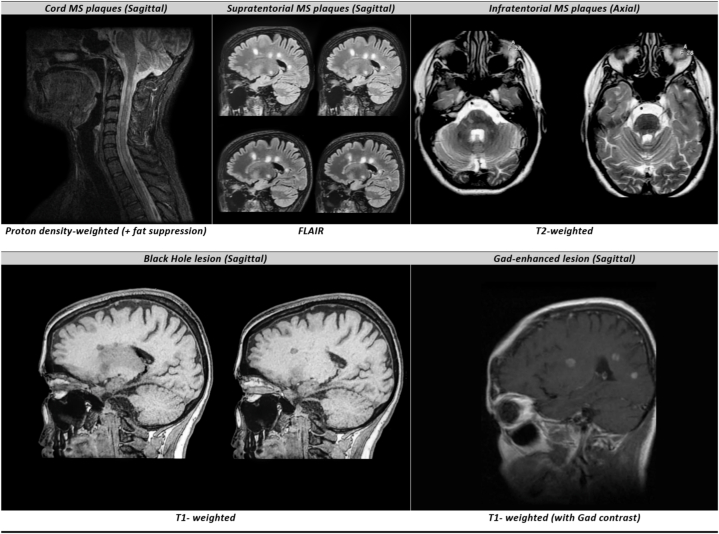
Different types of evaluated MRI plaques and lesions in this study (participants consented to have these images published); Abbreviations: Gad = Gadolinium.
